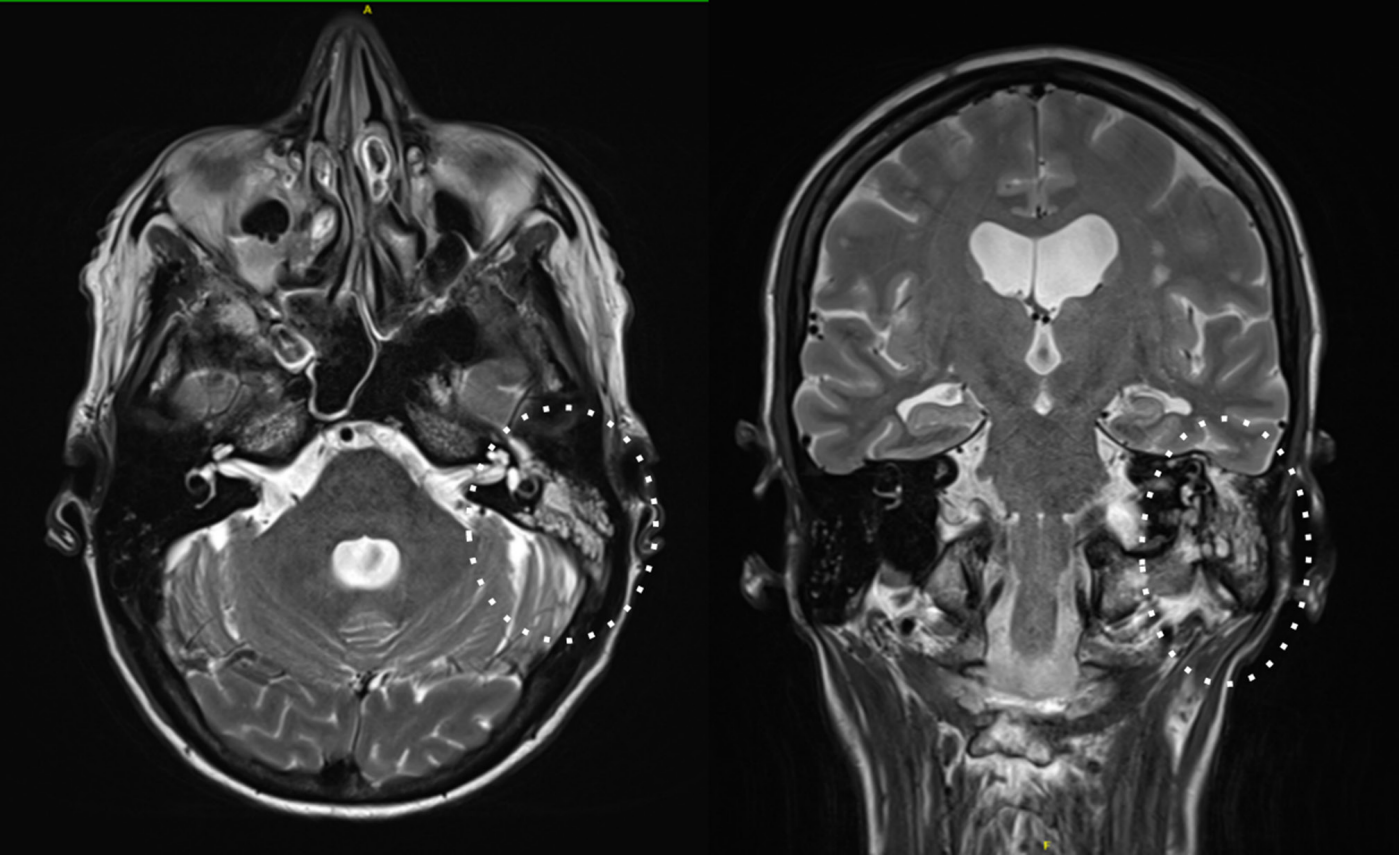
Left otomastoiditis on the patient’s MRI brain scan.
